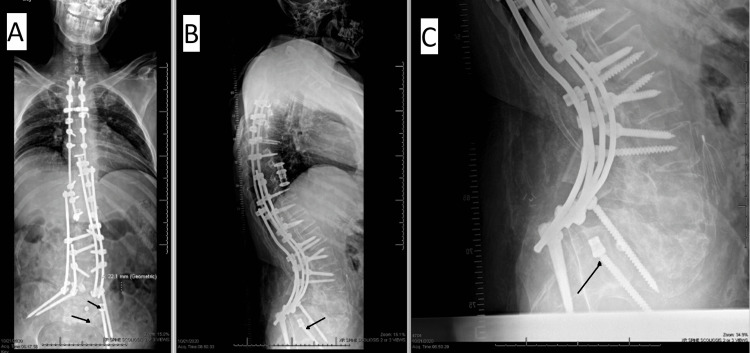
Radiographs demonstrating the lumbopelvic dissociation injury 26 days after the initial operation performed. A: Anteroposterior radiograph of the entire spine and construct. The short, superior arrow denotes the dislodged pelvic screw, and the long, inferior arrow denotes the inferior margin of the fracture line. The superior region of the pelvic fracture line can also be seen with the white dotted line. B: Lateral radiograph of the entire spine with the arrow denoting the pelvic screw that dislodged from the rod construct. C: Lateral radiograph of the lumbopelvic dissociation with the arrow again denoting the dislodged pelvic screw.
